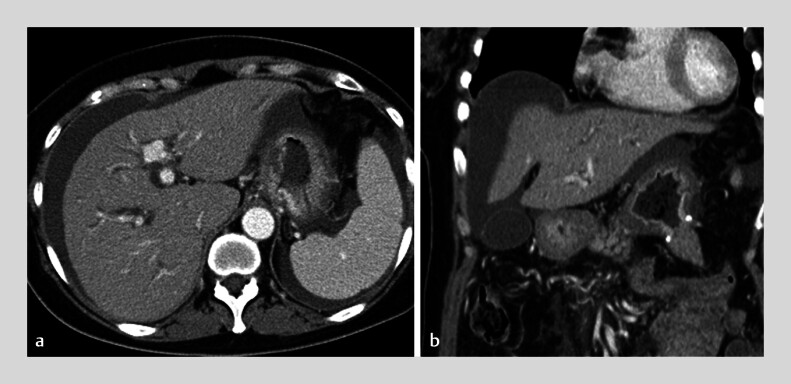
Preoperative computed tomography scan showing ascites present on the surface of the liver and between the stomach and liver.
